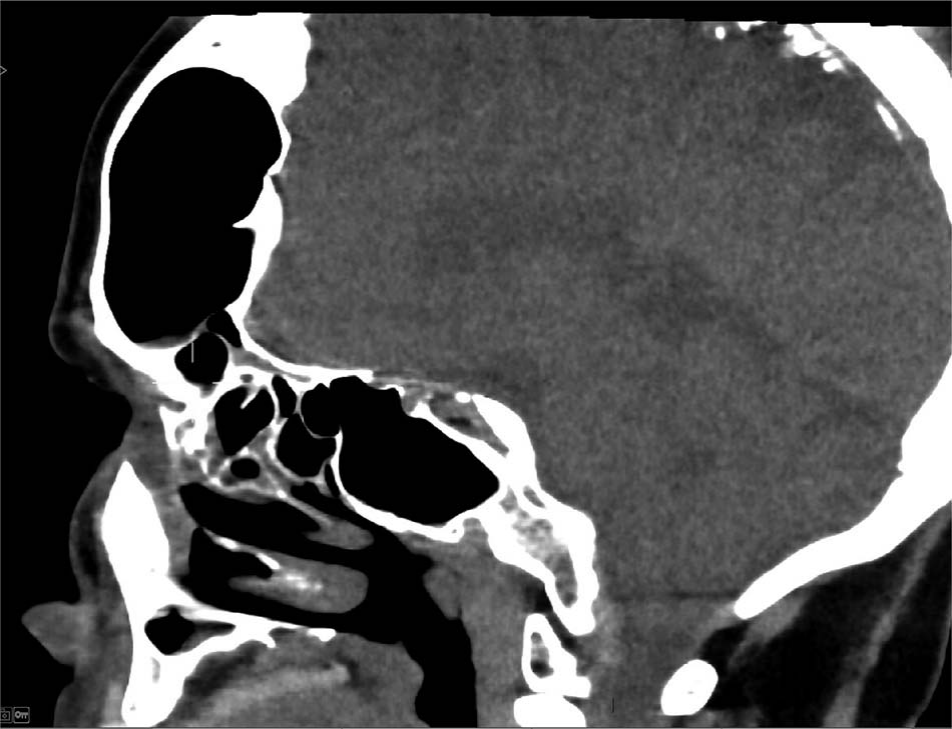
CT scan axial view showing hyperaeration of the left frontal sinus.
